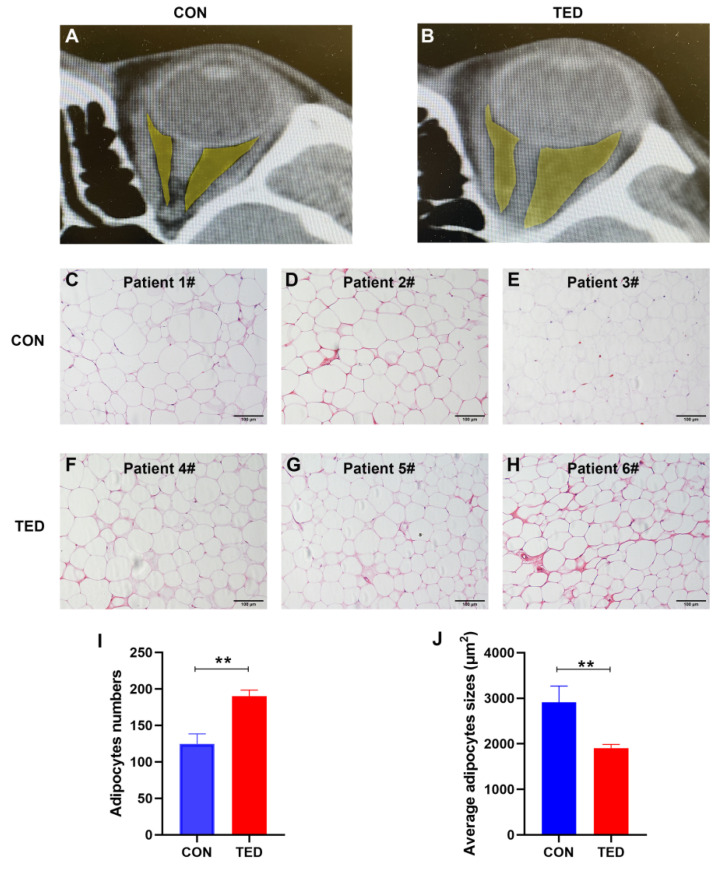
Hyperplasia rather than hypertrophy contributes to the enlargement of orbital adipose tissues (OATs) in thyroid eye disease (TED). Representative images of ocular computed tomography of patients with TED (A) shows an increased volume of OATs (indicated by yellow color) compared with the controls (B). Representative photographs (20×) of OATs derived from controls (C–E) and patients with TED (F–H) are shown. (I) In the quantification of adipocyte numbers, four fields were randomly selected from each section at a constant magnification (20×), and their average was calculated. There were more adipocytes in sections from TED-derived OATs (190.0 ± 7.6 cells/field) as compared with the controls (124.7 ± 11.3 cells/section). (J) Quantification of adipocyte sizes showed that TED-derived OATs had smaller adipocytes (1904 ± 72.34 μm^2^/cell, n = 1000 cells) compared with controls (2908 ± 293.9 μm^2^/cell, n = 1000 cells).  *p* < 0.01 as compared to the controls.
